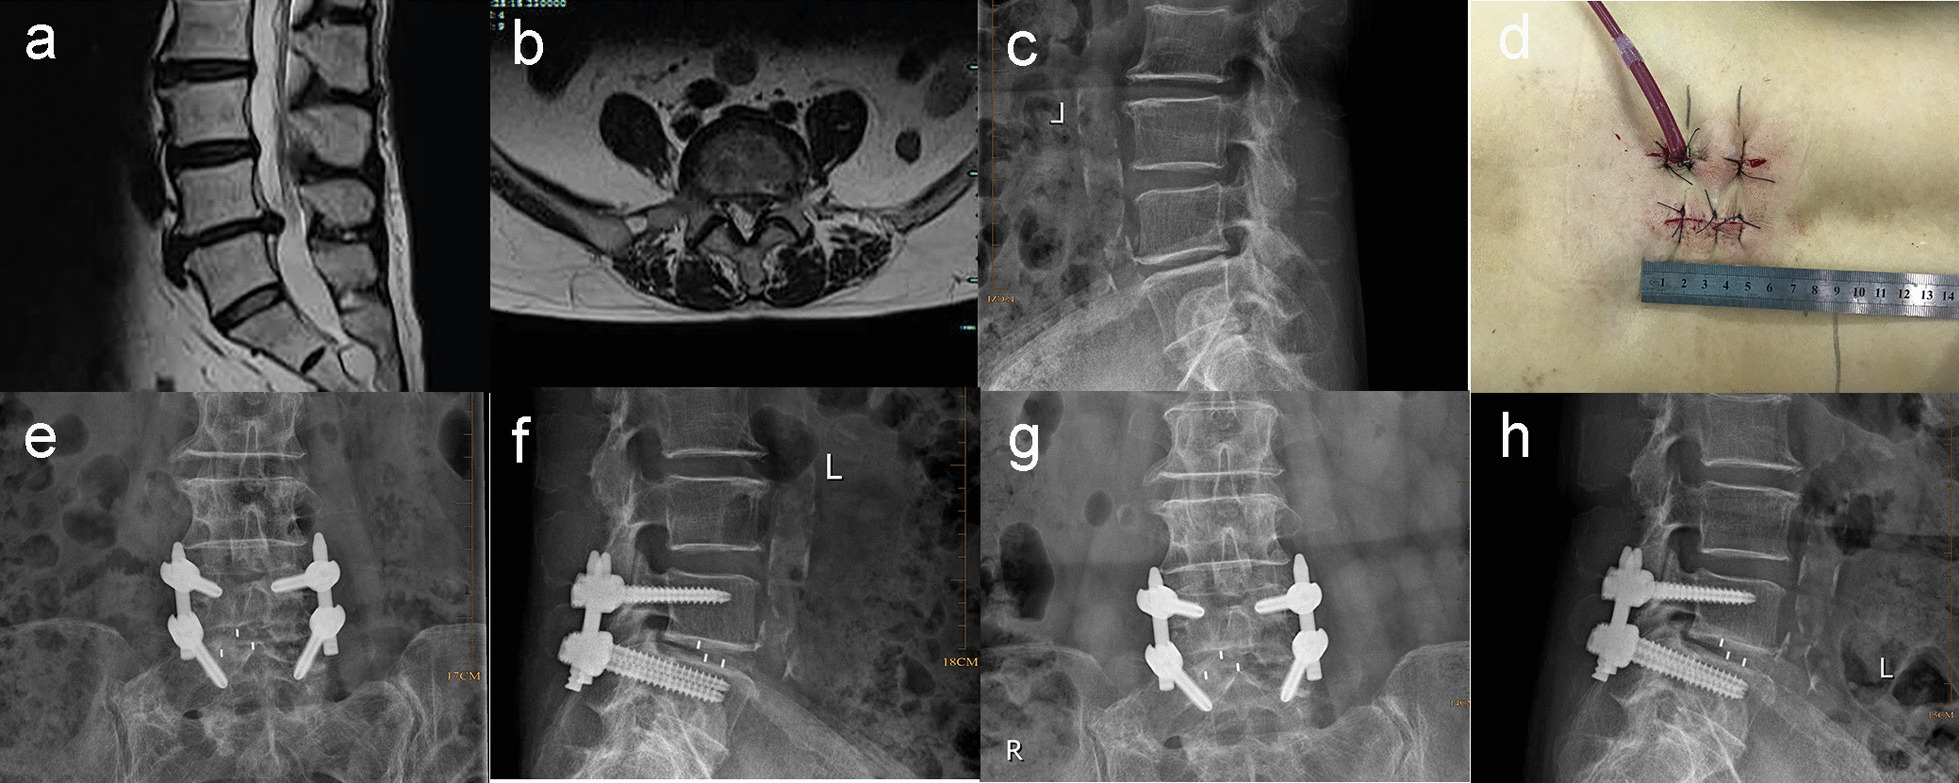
Typical case 1. A 66-year-old female with low back pain and left lower limb radiating pain for 2 years, aggravating for 6 months. Sagittal and axial MRI show disc herniation at L4/5 level (a, b). Lateral X-ray demonstrates reduced intervertebral height at L4/5 level (c). Postoperative surgical incision (d). Anteroposterior and lateral X-ray one month after surgery (e, f). Anteroposterior and lateral X-ray 1 year after surgery (g, h)
